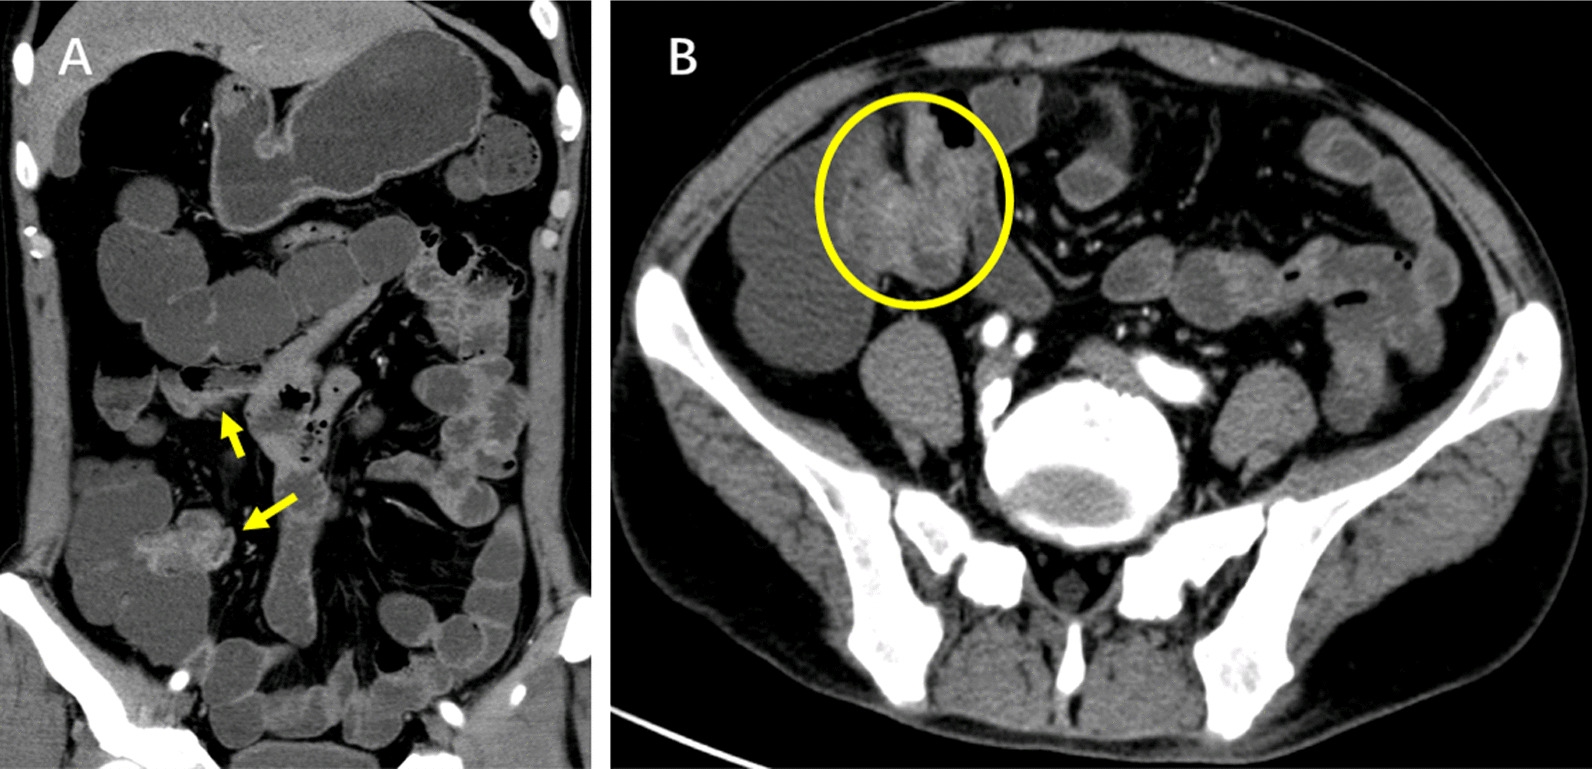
The coronal images (A) and cross-sectional images (B) of computed tomography enterography showed bowel wall thickening and mural hyperenhancement of terminal ileum and cecum (the arrows)
